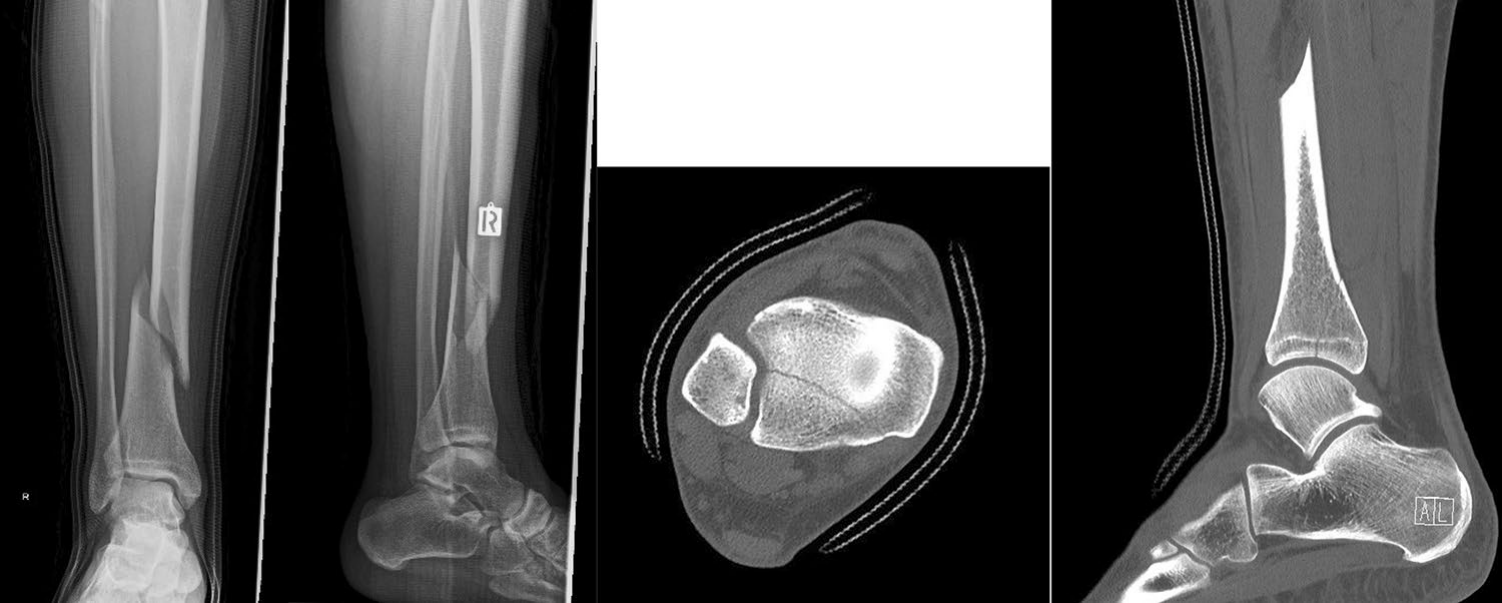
The figures show an example of the X-ray and CT images of a patient with a tibial shaft spiral fracture type A with fracture of the posterior malleolus. CT images were not available for the examiners. Additional screw osteosynthesis of the posterior malleolus was performed in this patient
